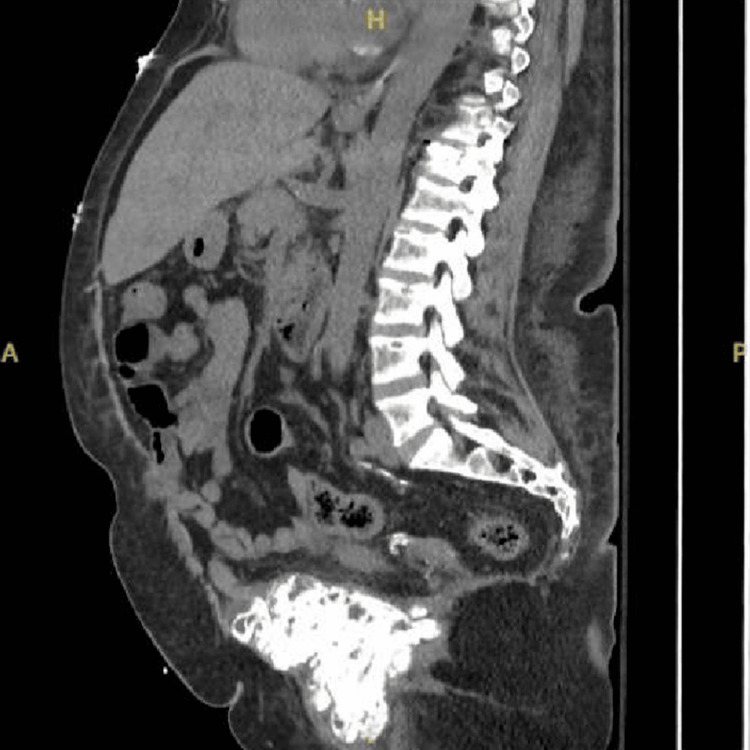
CT sagittal view of the abdomen and pelvis. Sagittal view of pubic uremic tumoral calcinosis, with the left worse than the right and a vertical measurement of 62.5 mm. Lytic changes of the spine and T12 fracture were also re-demonstrated as a result of renal osteodystrophy.
